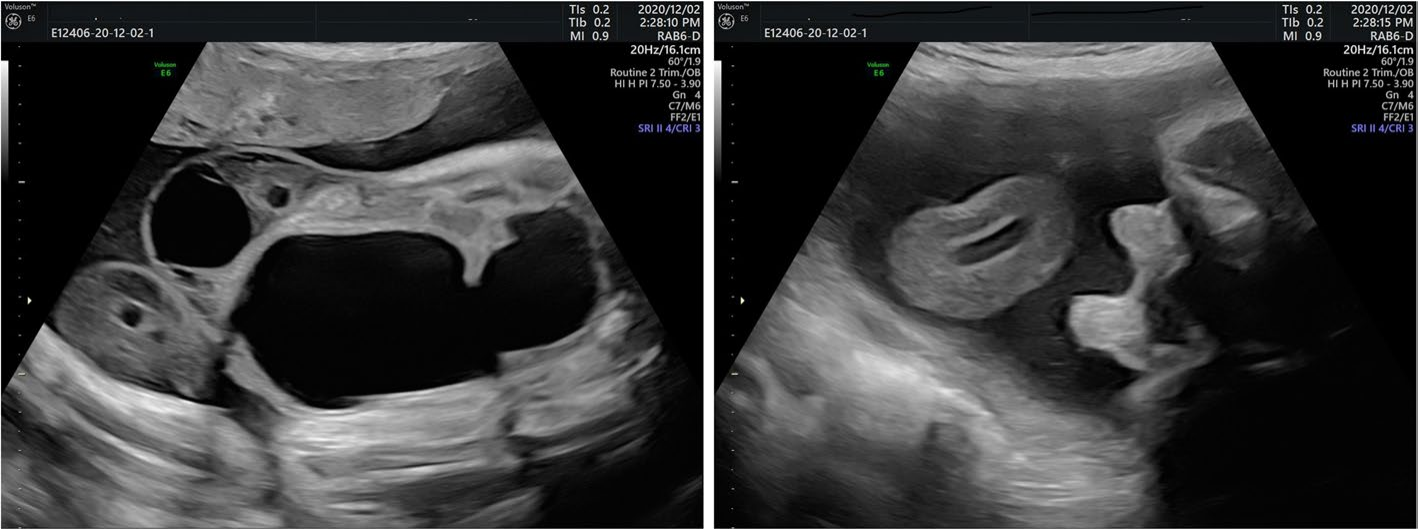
Ultrasound image at 32 weeks; large cystic structure disappeared. Large abdominal wall defect clearly seen
